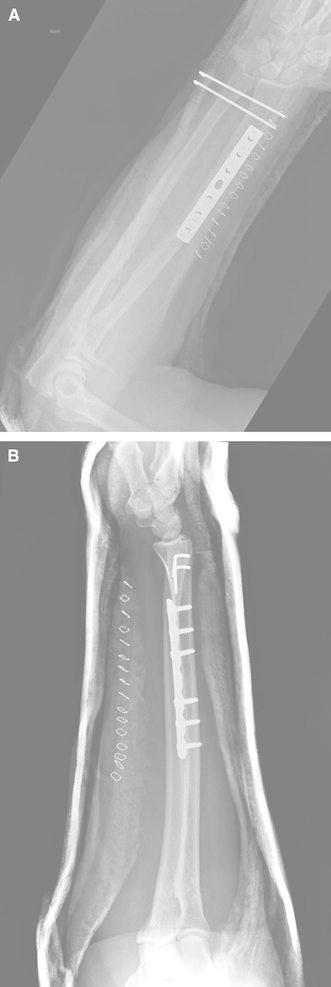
a AP forearm radiograph after surgical fixation and pinning of the DRUJ. b Lateral forearm radiograph after surgical fixation and pinning of the DRUJ
